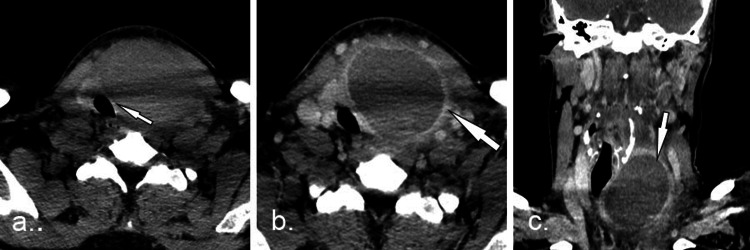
CT images of the patients neck (a) Axial non-contrast images showing a mass in the left infrahyoid neck displacing the trachea to the right (white arrow). (b) Axial contrast-enhanced images demonstrating ring enhancement of the lesion (arrow) with surrounding edema. (c) Coronal images depicting the abscess (arrow).
